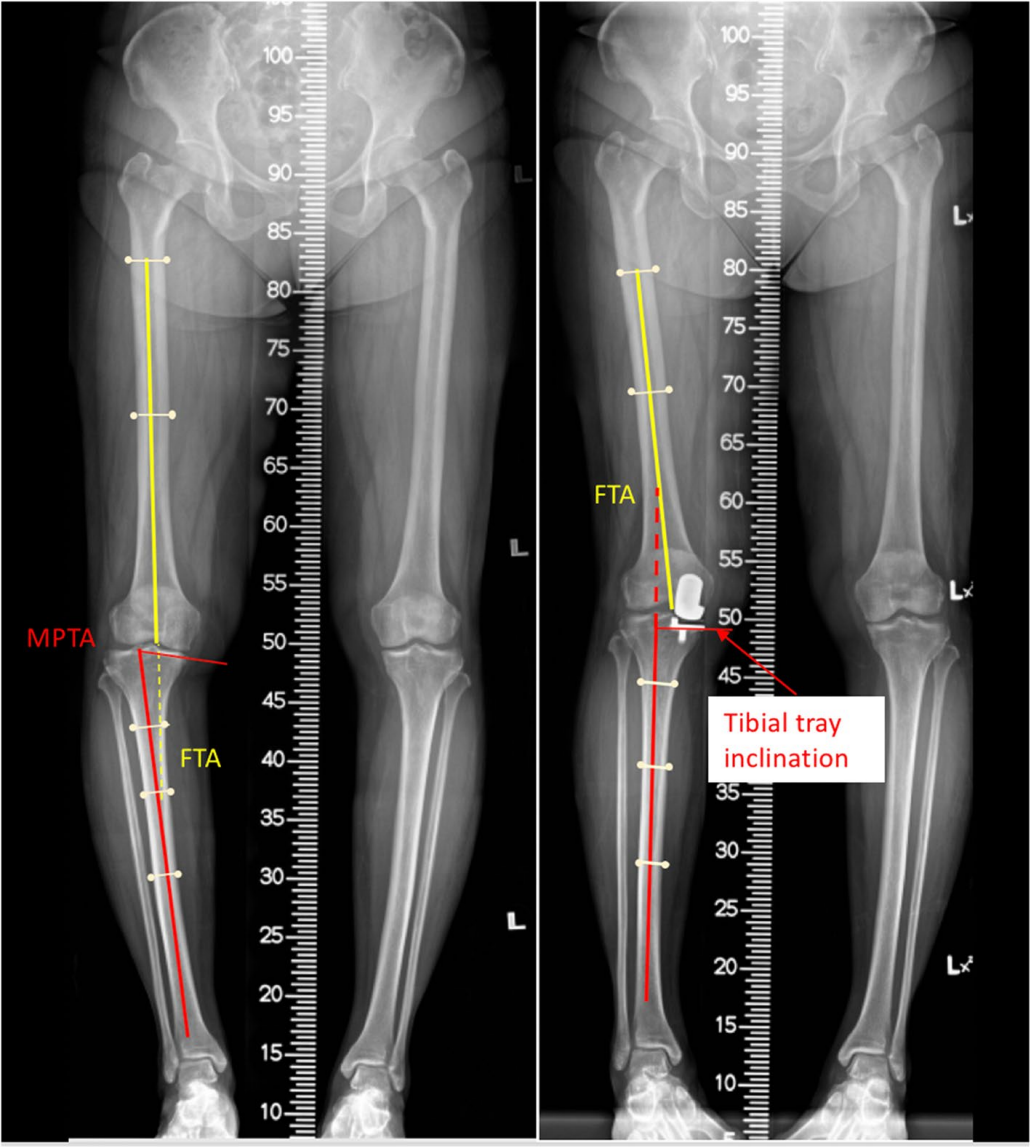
Mechanical femorotibial angle (FTA) and medial proximal tibial angle (MPTA), and tibial tray inclination were measured similarly. (Left) preoperative and (Right) postoperative radiographic measurement
